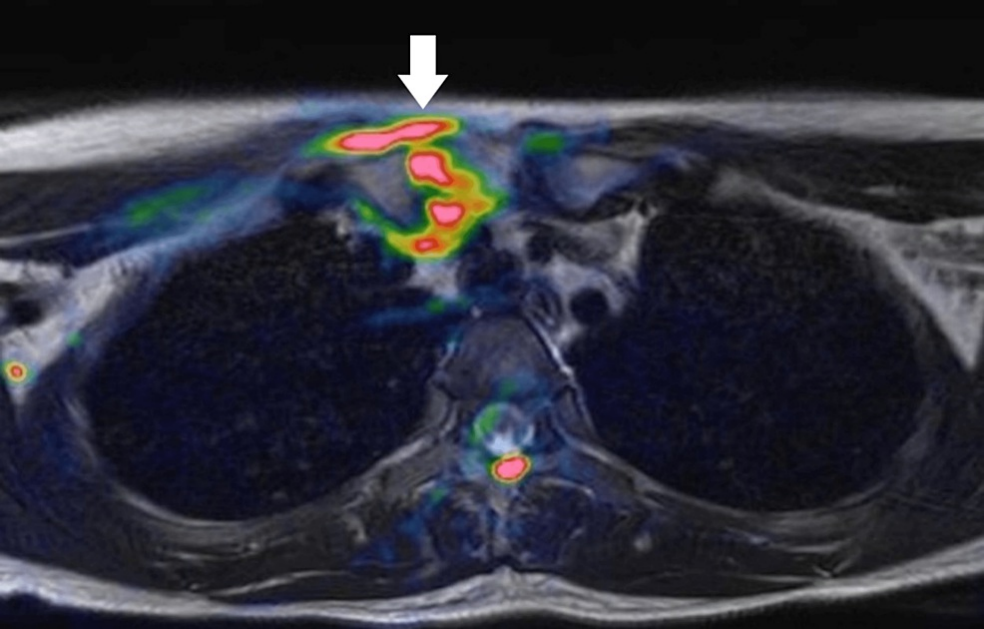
MRI showing a high signal intensity area around the right sternoclavicular joint (arrow) on the diffusion-weighted image (fusion T2-weighted image).
